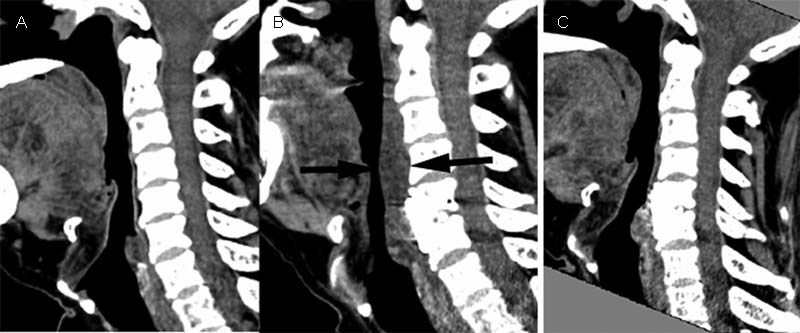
Sagittal reconstruction of cervical computed tomography images: (
